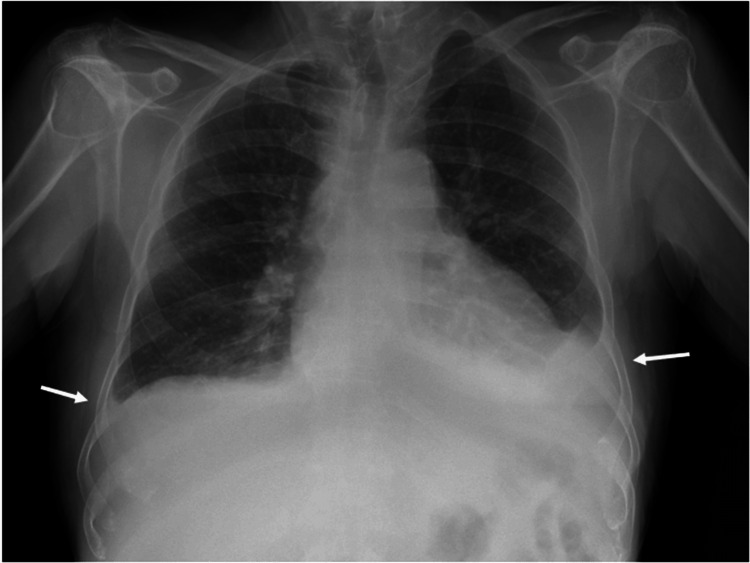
Bilateral pleural effusion visible on the thoracic X-ray. The arrows point to the pleural effusion.
